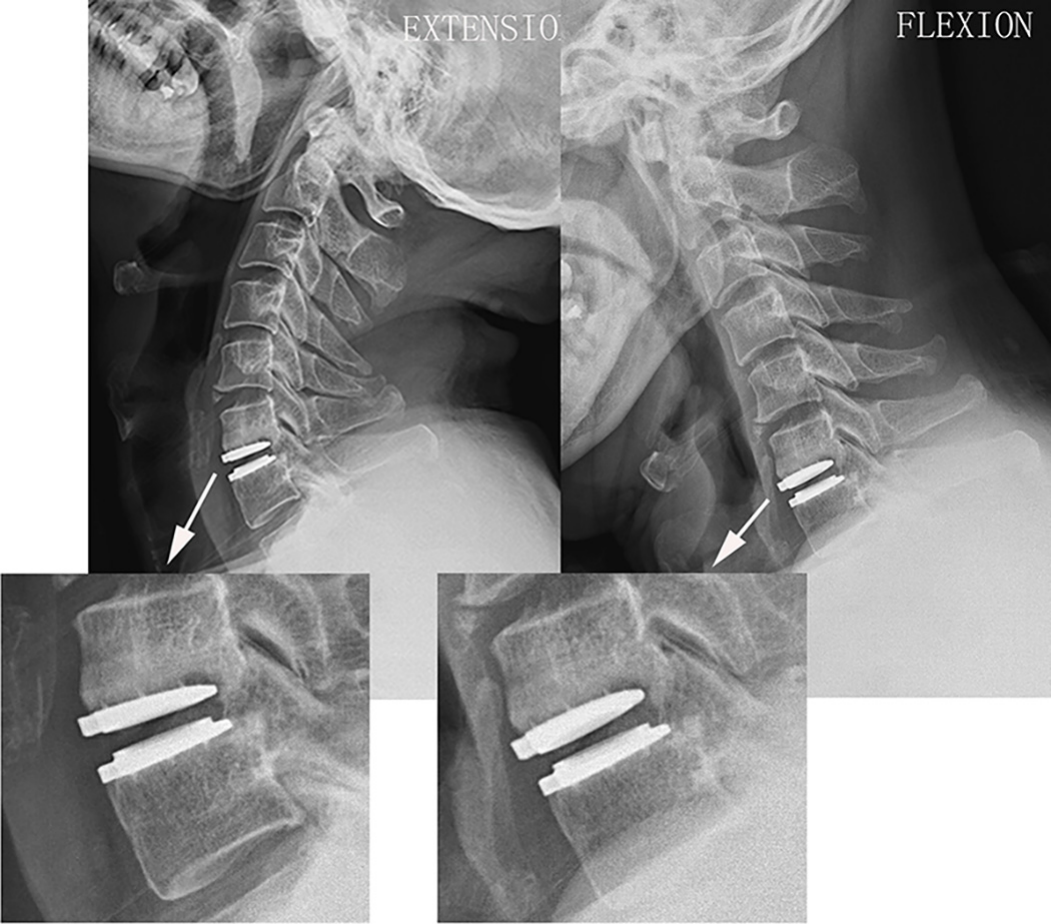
The representive case who suffered heterotopic ossification of Discover prosthesis postoperatively. Dynamic flexion-extension lateral radiographs at the final follow-up showing heterotopic ossification (Grade III) at the index level with significant restriction in the range of motion of Discover prothesis.
